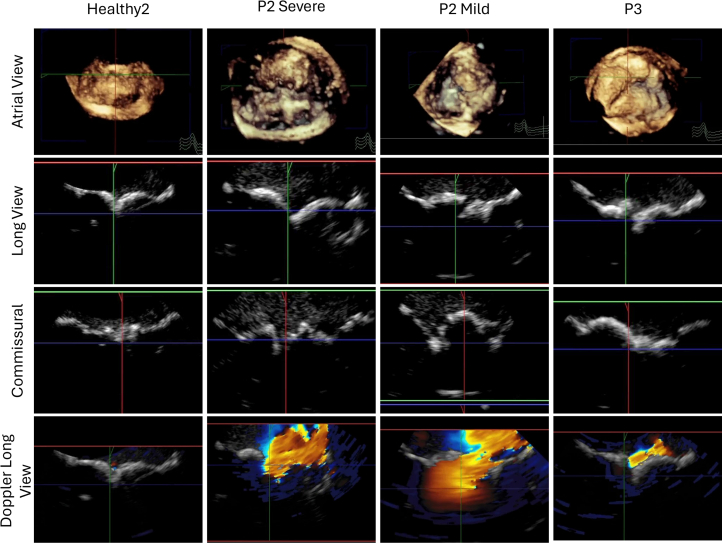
Standard axial images collected with transesophageal echocardiography probe of the Healthy2 valve (mean healthy dimensions) and each of the prolapse valve models (*columns*). Rows show axial view, long-axis, and commissural b-mode views, and a long-axis Doppler view for each valve.
